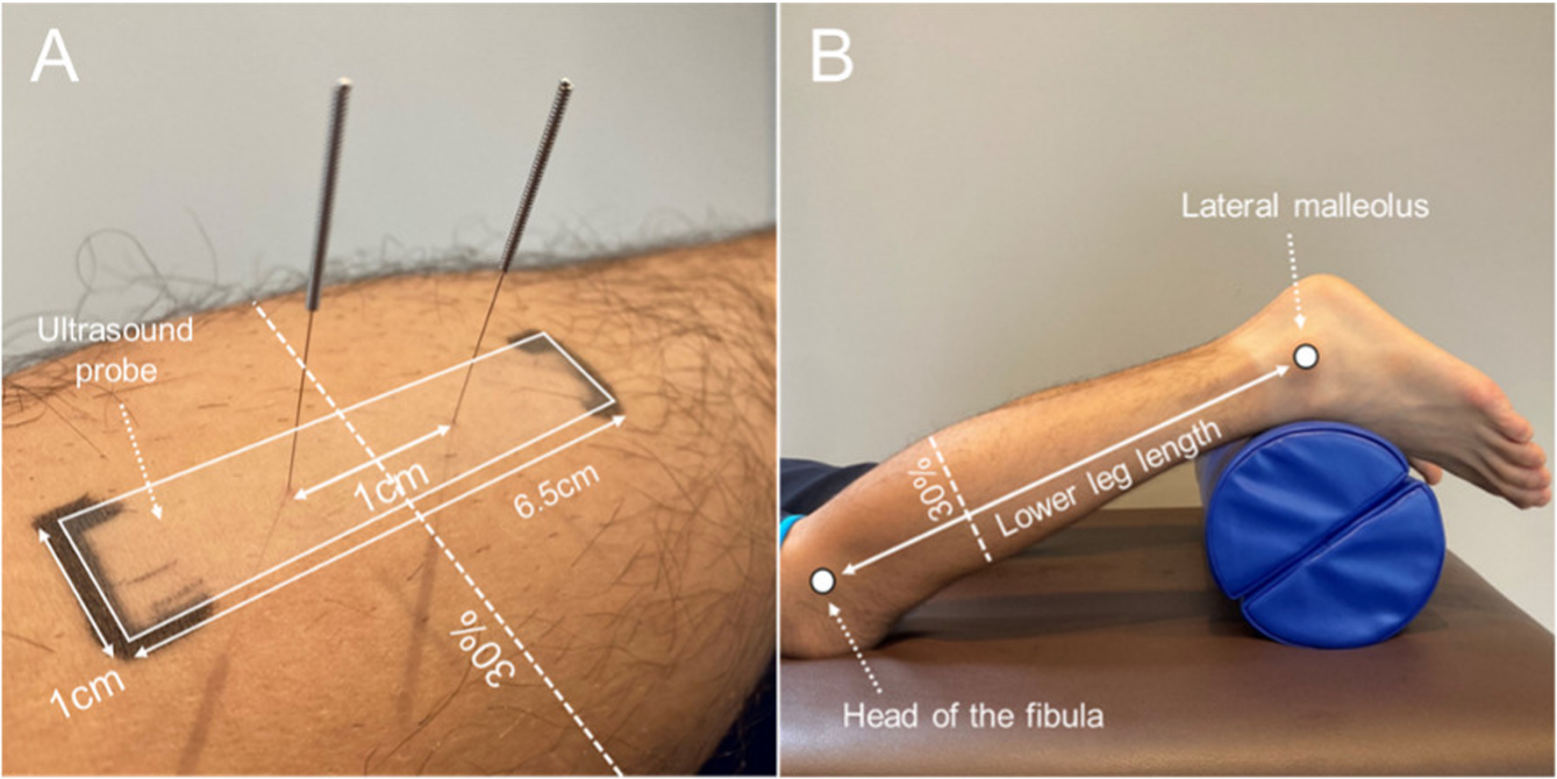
(A) A marker (6.5 cm × 1.0 cm) aligned with the ultrasound probe placed at the proximal 30% site of the lower leg length (lateral knee joint cleft to external capsule). (B) Lower extremity position during measurement and low-frequency electroacupuncture.
